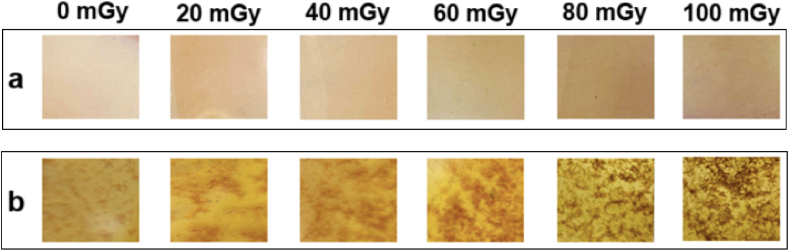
Color change in PVA/NBT films for two different concentrations of NBT dye a: 1 g/L, b: 10 g/L, after being exposed to various X-ray doses.
